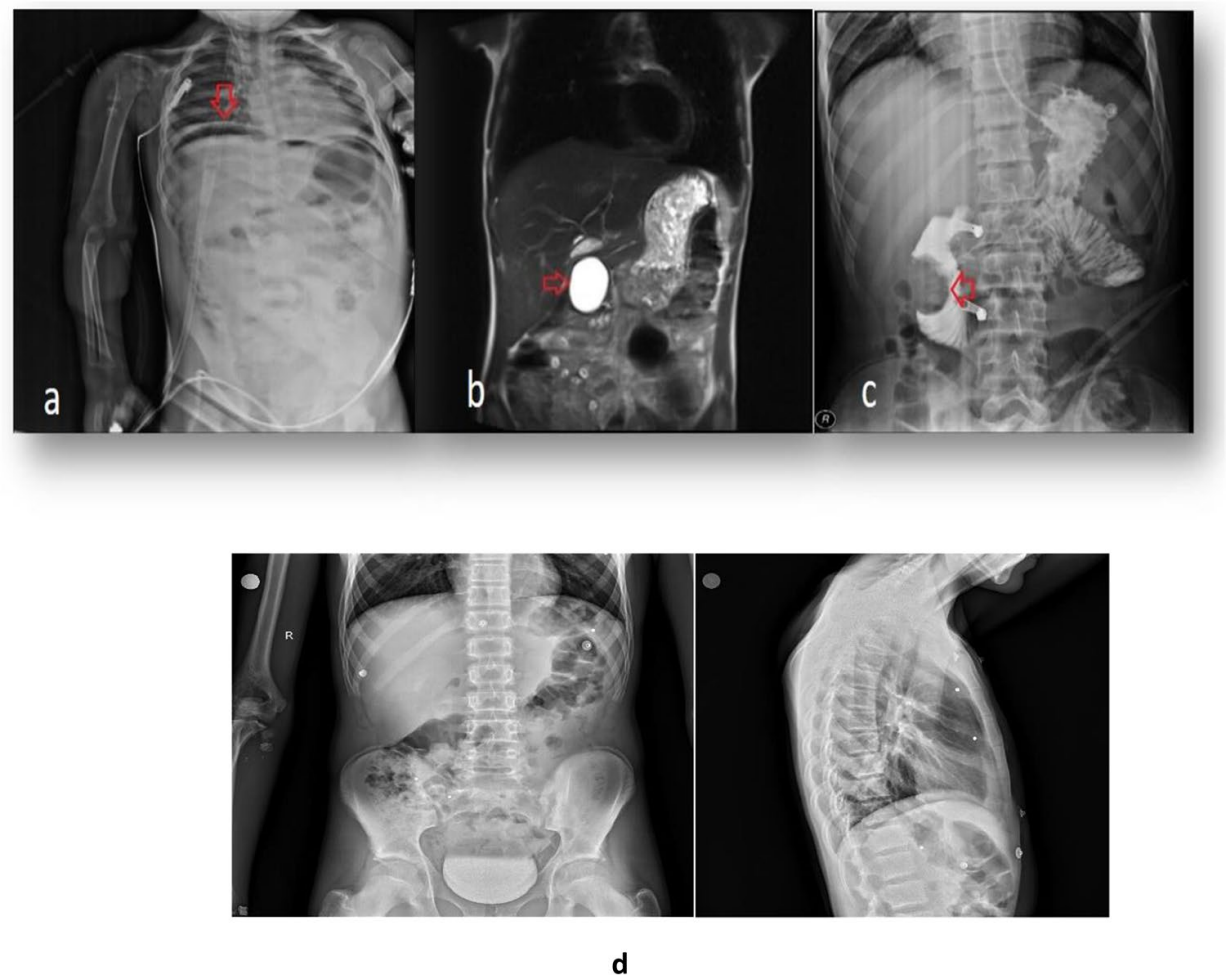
Various radiological images of pancreaticoduodenal trauma cases. a Duodenal perforation, free air under the right diaphragm on a direct radiograph (arrow). b The pseudocyst’s image on abdominal MRI (arrow). c Duodenal hematoma filling the lumen on gastric-duodenal contrast radiography (arrow). d Direct anteroposterior and lateral X-ray images of the patient with pancreatic trauma due to gunshot injury (radiopaque pellets are observed)
